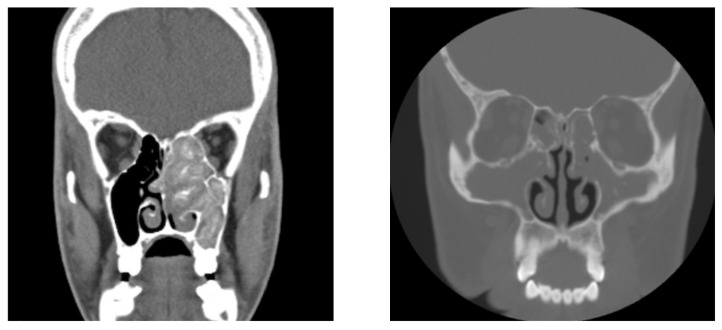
(Left) Coronal CT of allergic fungal rhinosinusitis, with opacification and expansion of paranasal sinuses. (Right) Coronal CT scan of paranasal sinuses with features consistent with chronic rhinosinusitis, with opacification and possible bony erosion consistent with invasive infections. Images courtesy of the Otolaryngological and Radiological Departments of Heliodor Święcicki Hospital.
